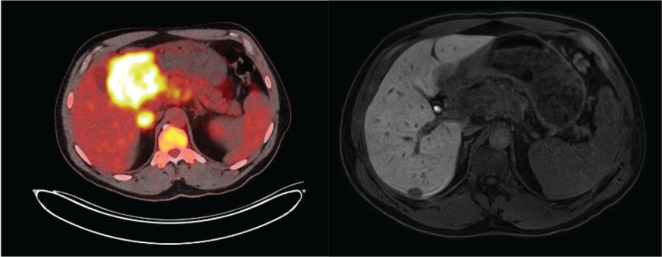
Preliminary staging with PET scan (left) and liver MRI (right). The PET scan shows a clear uptake of the primary tumor with regional lymph nodes. On MRI, a suspicion of liver metastasis is seen just at the junction between segments V and VI.
